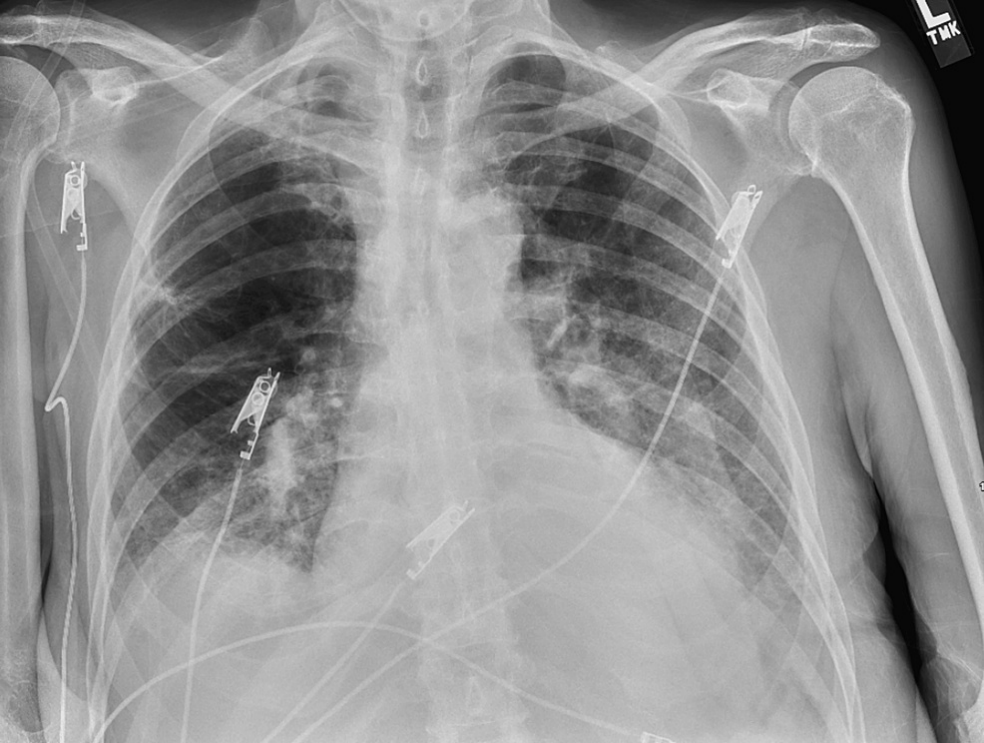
Chest X-ray showing interstitial thickening with ground-glass density in both lower lobes.
